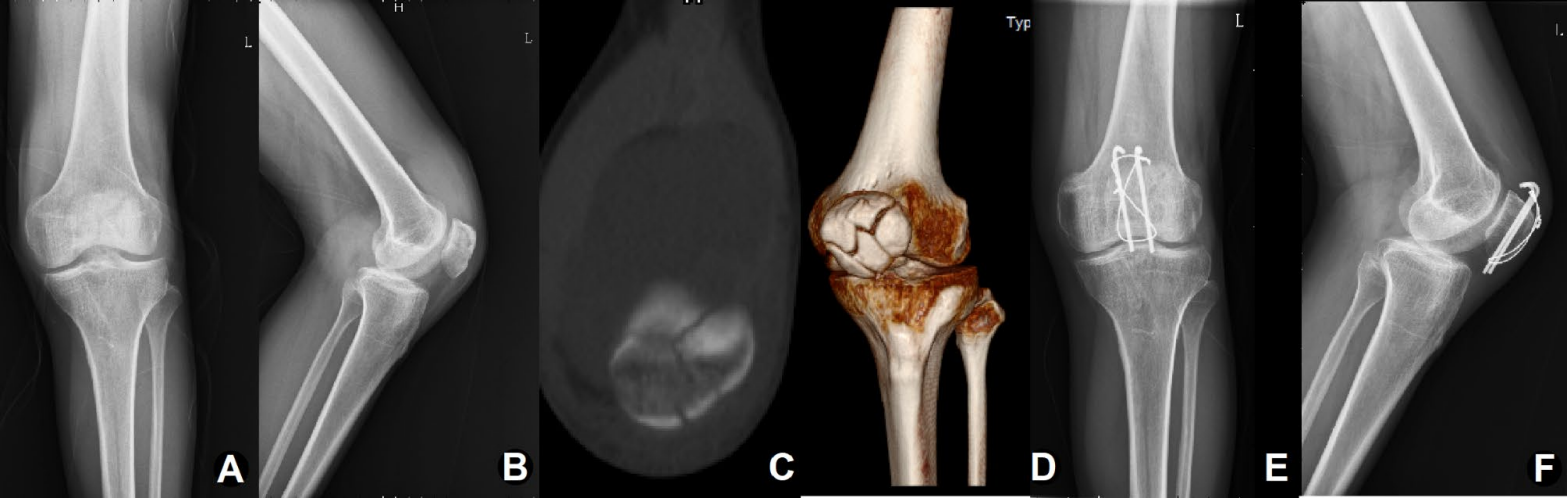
Case example of comminuted patellar fracture (Male, 43 years old, left side) using the suture reduction combined with the modified K-wire tension band. (A, B) Preoperative X-ray indicated displaced comminuted patellar fractures. (C, D) The regular follow-up at 1.5 years after operation showed fracture union without displacement of internal fixation and (E, F) the internal fixation was removed two years after surgery
